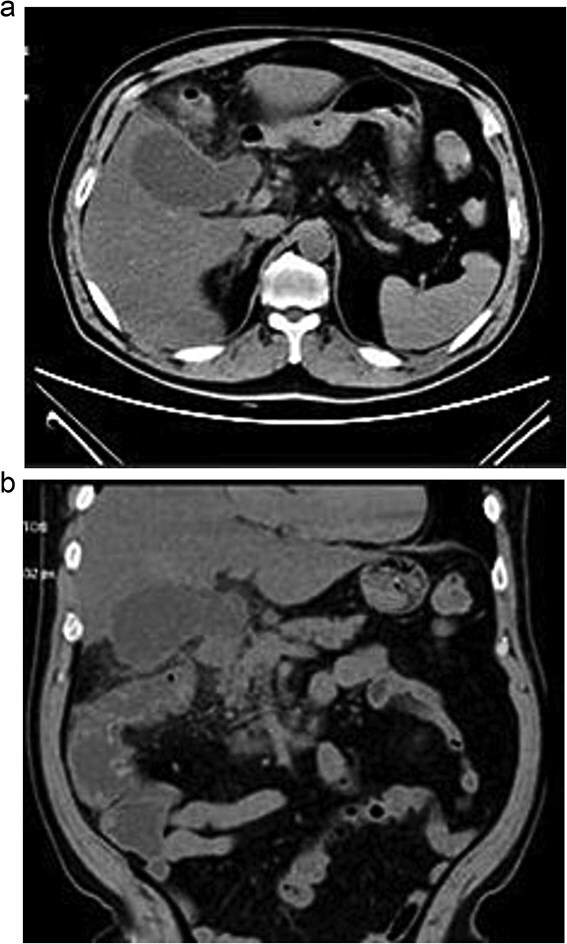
Axial (a) and coronal (b) abdominal CT showing gallbladder hydrocholecystosis.
